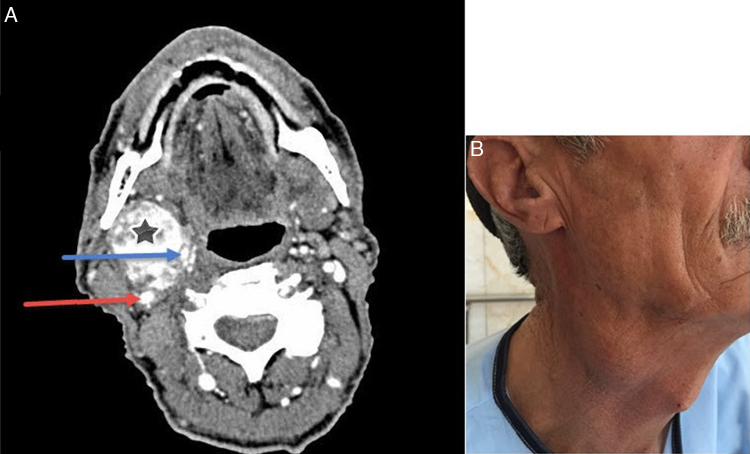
(A) Spiral neck CT scan with IV contrast shows CBT (star), ICA (red arrow) and ECA (blue arrow). (B) A 60 year-old man with a painless, non-pulsatile right neck mass since 40 years ago.
